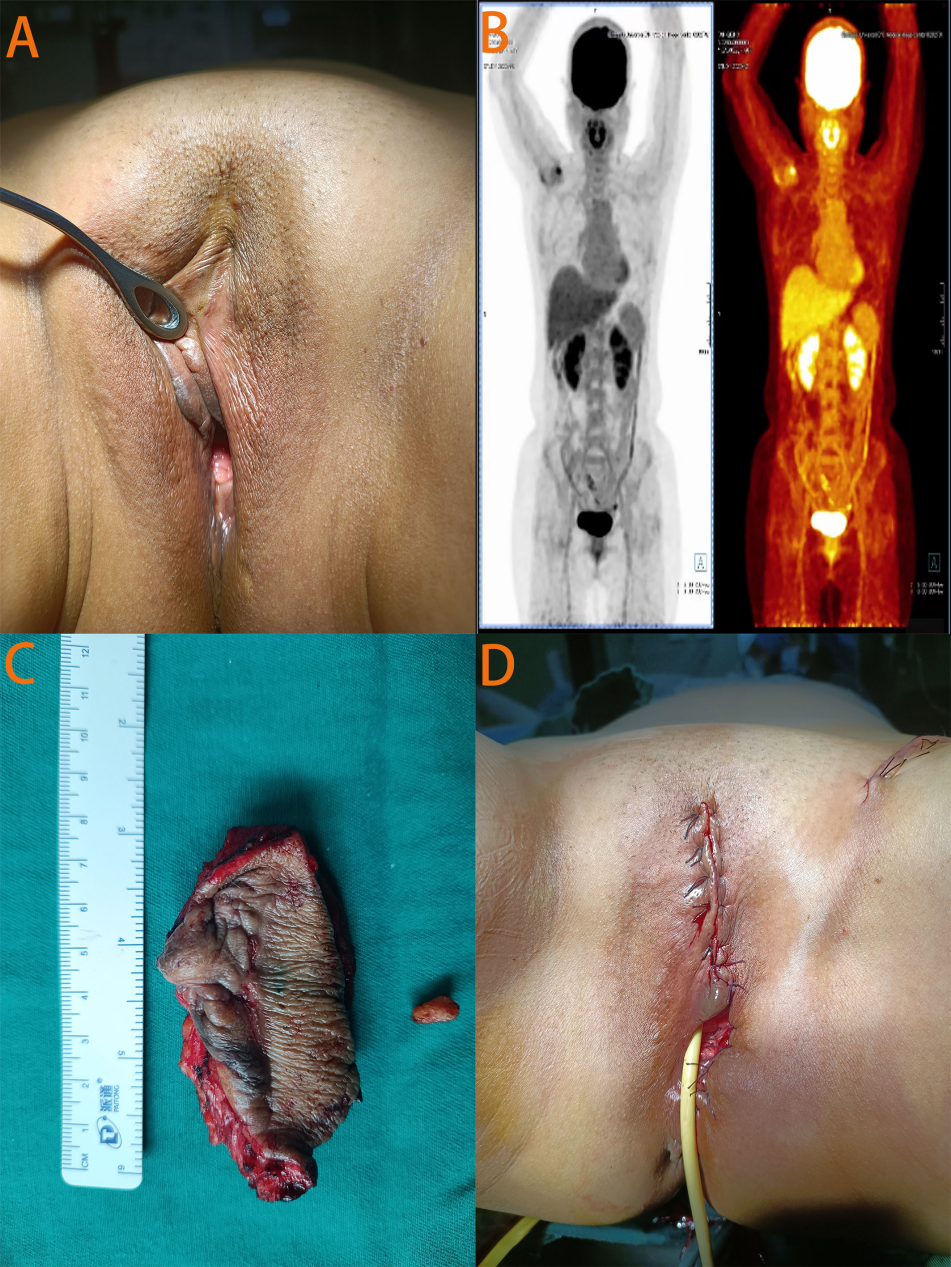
Relevant auxiliary examination images and pre - and post-operative photos of the patient. (A) In the photo taken before surgery, a scar about 1cm in size was visible at the primary site of the disease. (B) The PET-CT images showed no evidence of disease elsewhere. (C) The surgically excised left vulvar tissue and left inguinal lymph node. (D) Photograph taken at the end of the surgery.
